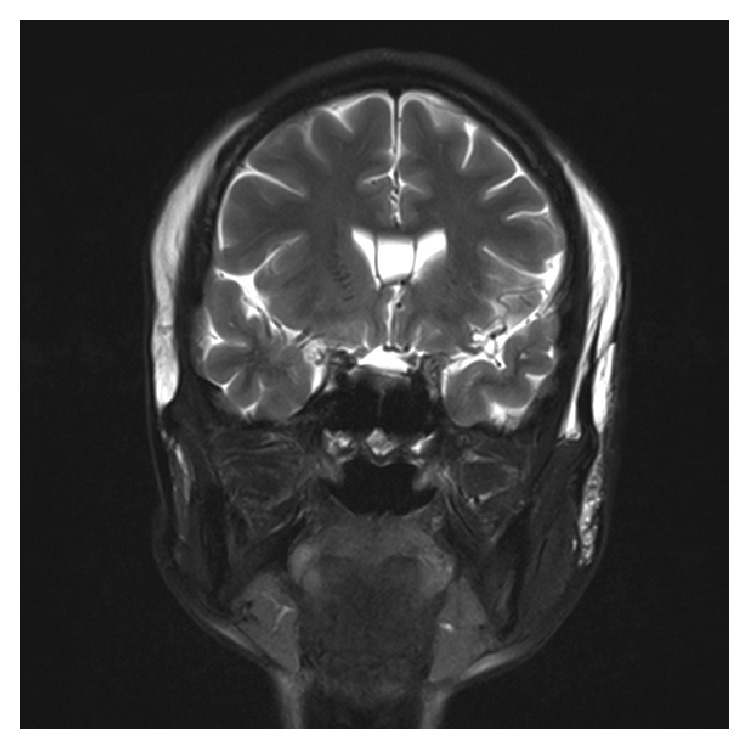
Coronary MR image showing PAAG migration into the cheeks and neck.
